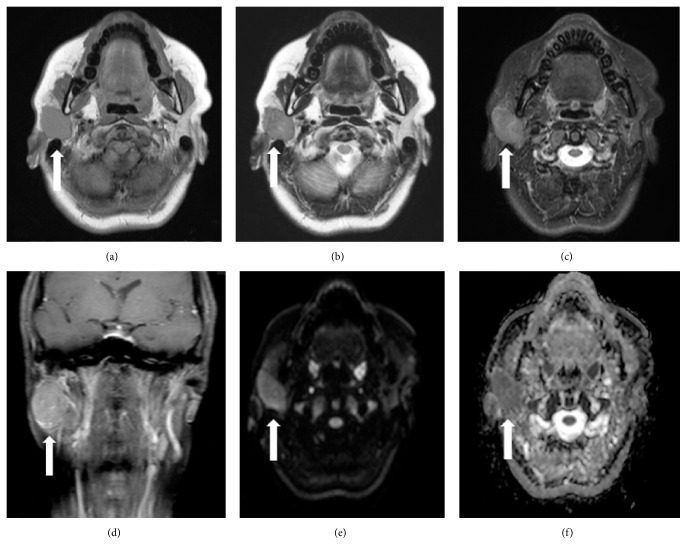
Magnetic resonance images of parotid oncocytoma. (a) T1-weighted imaging. (b) T2-weighted imaging. An axial magnetic resonance image shows a well-demarcated mass (white arrow) in the superficial and deep lobe of the right parotid gland. (c, d) The mass appears hyperintense to the native parotid gland tissue on fat-saturated T2-weighted imaging but hypointense on contrast-enhanced, fat-saturated T1-weighted imaging. (e, f) On diffusion-weighted images, the mass appeared hyperintense and hypointense according to the apparent diffusion coefficient.
